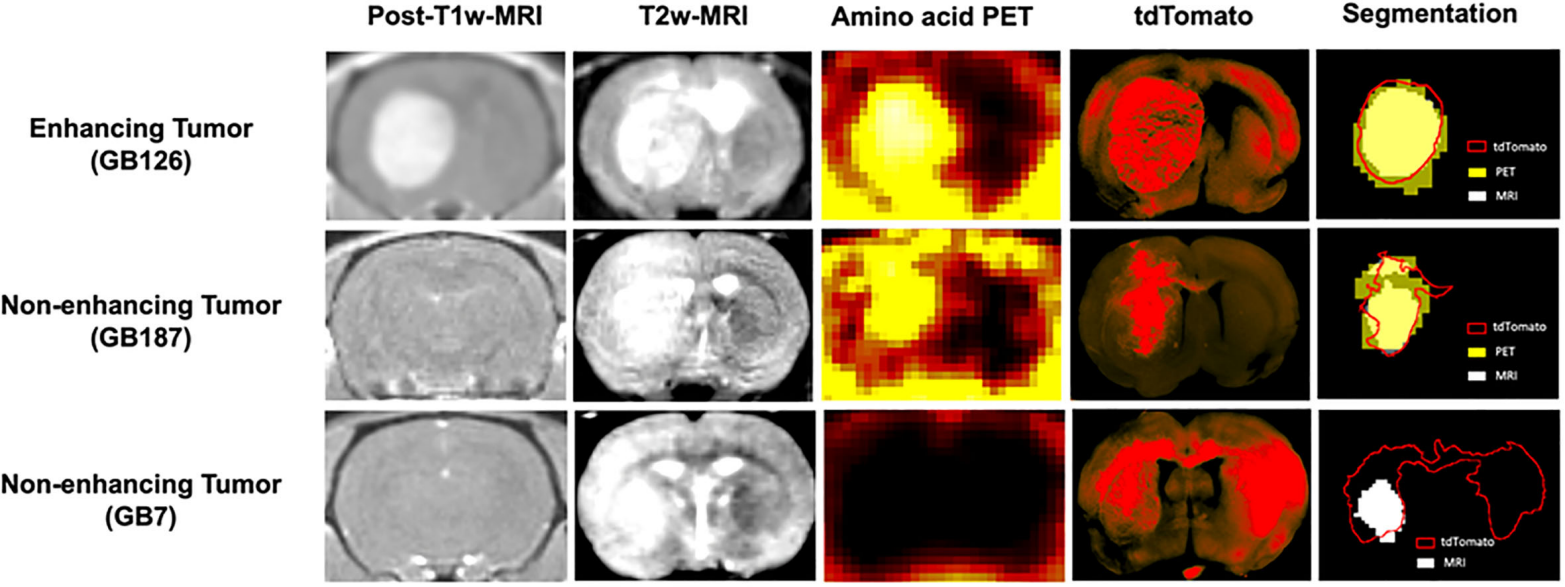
Coronal brain images showing post contrast-T1-weighted-MRI, T2-weighted-MRI, amino acid PET, tdTomato optical imaging, and tumor segmentations. The top row shows a gadolinium enhancing tumor (GB126). The middle row shows a gadolinium non-enhancing tumor detected with PET (GB187). The bottom row shows a gadolinium non-enhancing tumor not detected with PET (GB7).
